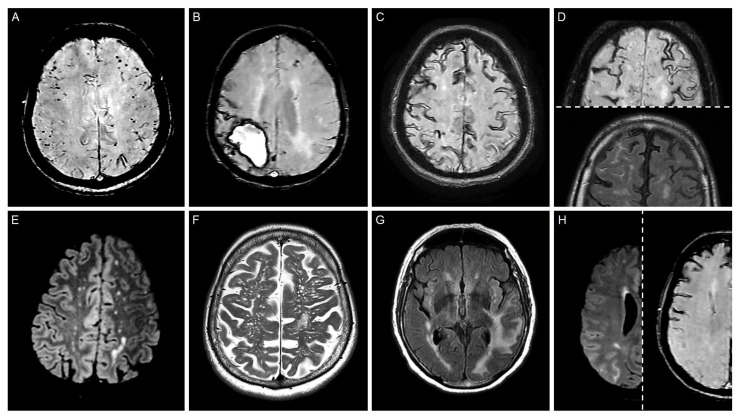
Hallmark magnetic resonance imaging features of CAA and CAA-RI. (A) Strictly lobar CMBs presenting as small globular hypointensities on SWI (a left parietal CSS can also be appreciated). (B) Lobar ICH (late subacute) in the right parietal lobe on SWI (several surrounding CSS, a small left parietal cSS, and two left frontal CMBs can also be observed). (C) Disseminated CSS in form of gyral hypointensities on the cortical surface on SWI in a patient presenting with TFNEs (a single CMB can also be appreciated right postcentrally). (D) Multiple foci of cSAH on the right prefrontal convexity presenting as a constellation of SWI hypointensity filling the affected sulci with corresponding SNN (i.e., sulcal hyperintensity) on the FLAIR (multiple chronic cSS can also be appreciated with no corresponding SNN). (E) White matter hyperintensities in a multispot pattern on FLAIR in the bilateral subcortical white matter, a feature introduced in the Boston criteria v2.0 [106]. (F) Severe MRI-visible (i.e., dilated) perivascular spaces in the centrum semiovale on T2, a feature introduced in the Boston criteria v2.0 [106]. (G) Asymmetric confluent white matter hyperintensity corresponding to vasogenic edema (also known as. ARIA-edema) in the left temporal, parietal, and occipital lobes on FLAIR in a patient with strictly lobar hemorrhagic alterations and subacute cognitive decline, meeting the classical criteria for probable CAA-RI (foci of thin SNN can also be appreciated; the edema completely resolved after corticosteroid therapy). (H) Right parietal SNN without corresponding SWI hypointensity (corresponding to ARIA-effusion) in a patient presenting with TFNEs and disseminated cSS, meeting the proposed extended criteria for probable CAA-RI [154]. (ARIA, amyloid-related imaging abnormality; CAA, cerebral amyloid angiopathy; CAA-RI, CAA-related inflammation; CMB, cerebral microbleed; cSAH, convexity subarachnoid hemorrhage; cSS, cortical superficial siderosis; FLAIR, fluid-attenuated inversion recovery; ICH, intracerebral hemorrhage; MRI, magnetic resonance imaging; SNN, sulcal non-nulling; SWI, susceptibility-weighted imaging; TFNE, transient focal neurological episode).
